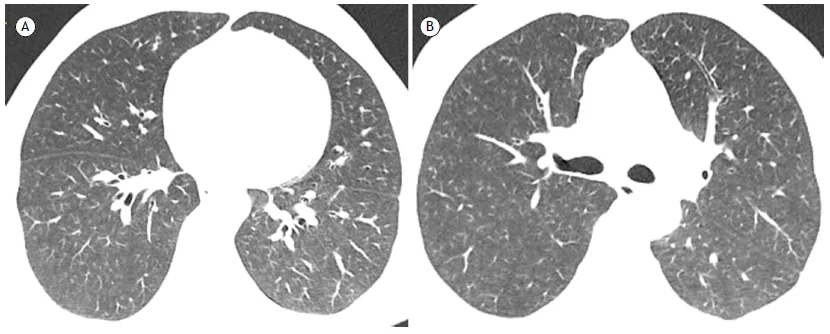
Follow-up chest HRCT scans showing resolution of the ground-glass micronodules after adalimumab discontinuation (in A and B).
